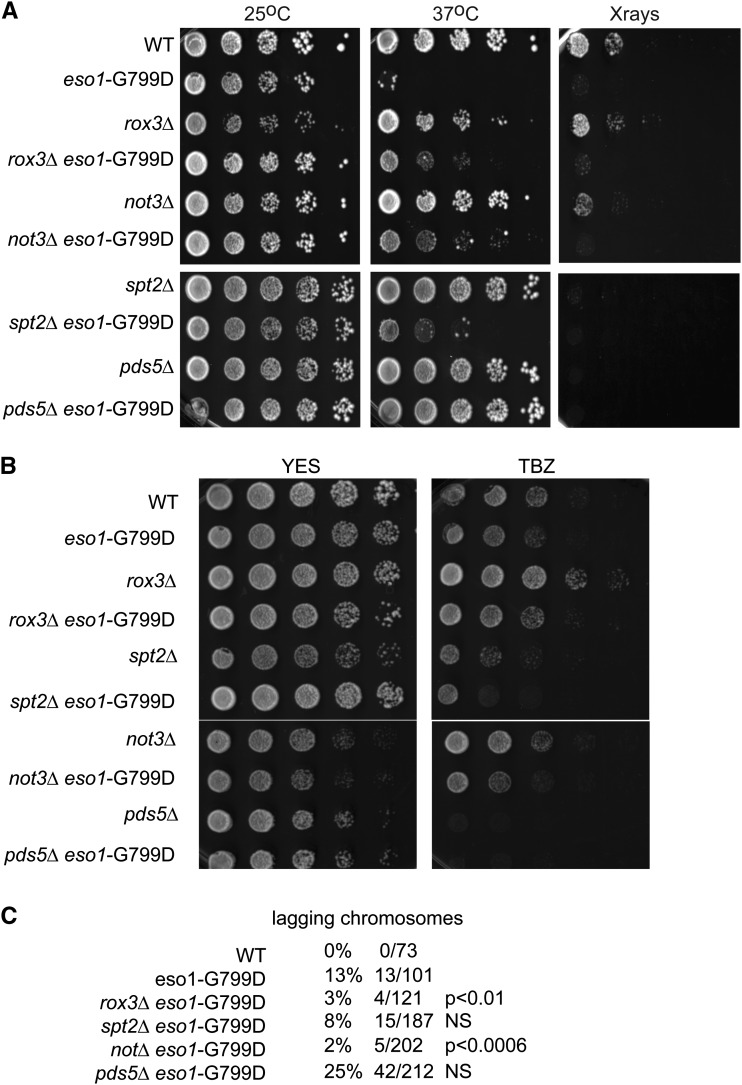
Deletions that rescue the *eso1*-G799D allele at nonpermissive temperature. Deletion of *rox3*, *not3*, and *spt2* can partially rescue the growth defect at 37° of *eso1*-G799D mutation. (A) *rox3*, *spt2*, and *not3* deletion can partially rescue the growth defect at 37° caused by *eso1*-G799D mutation. *pds5* deletion was used as the control. None of these deletions can alleviate the hypersensitivity of the *eso1*-G799D mutant to 75Gy X-ray treatment. Deletions that rescue the *eso1*-G799D allele at nonpermissive temperature do not rescue TBZ sensitivity (B), but show partial rescue of lagging chromosomes (C).
